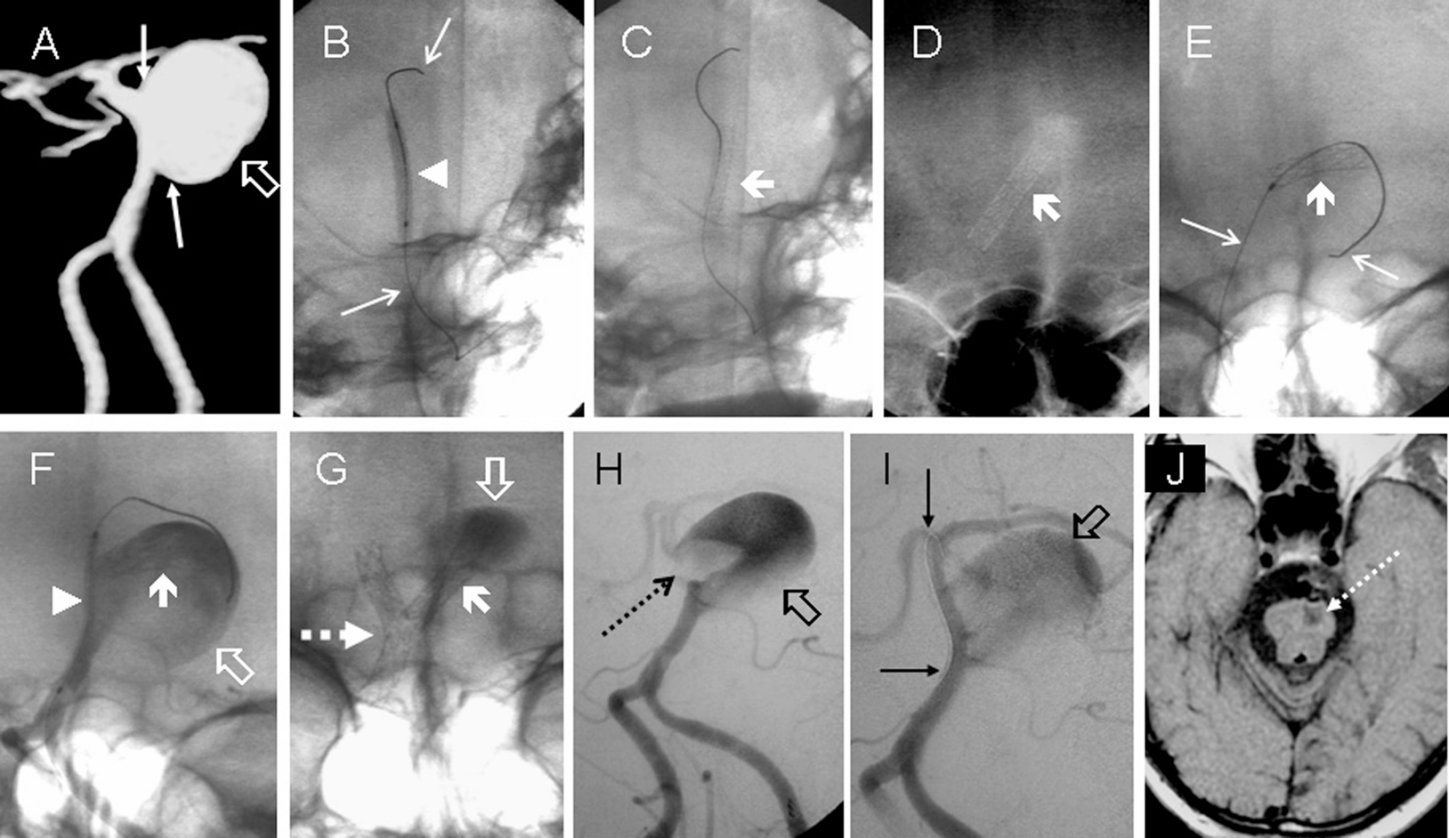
Imaging of the aneurysm before (A), during the stenting (B-G) and in vascular rescue procedure (H-J). A) Preoperative angio-CT: Aneurysm of the basilar artery (hollow arrow) and evidence of wide neck (thin arrows). B) to G) First procedure. A balloon-expandable stent (arrowhead in B and thick arrow in C) is advanced on a microguidewire (thin arrows in B). Once the stent was deployed, it slid out of place towards the sac (thick arrows in D). The whole misplaced stent was gently pushed into the sac (thick arrow in E and F) using a balloon mounted on the same microguidewire (thin arrows in E). A second balloon-expandable stent (arrowhead in F) is placed over the entire length of this wide-necked aneurysm (hollow arrow in F). This second balloon-expandable stent is in a correct position within the parent vessel (dotted arrow in G), the first misplaced BES lies within the aneurysm (thick arrow in G) and a sluggish intraaneurysmal vortex motion is clearly shown (hollow arrow in G). Second procedure. Neurological impairment four hours later explained by in-stent thrombosis. An intravascular clot initially emerging from the thrombosing sac (hollow arrow in H) overflows into the basilar artery (dotted arrow in H). Both pharmacological and mechanical measures using a microguidewire (thin arrows in J) successfully recanalize the arterial tree distal to the aneurysm (J). Imaging follow-up. At one week, the T1 magnetic resonance imaging shows a residual stroke in the ventral portion of the cerebral peduncle (dotted arrow in K).
